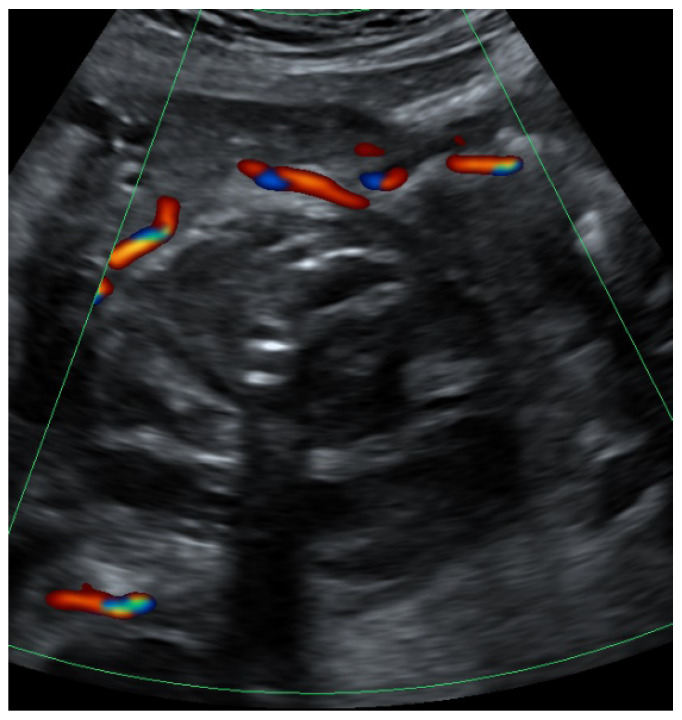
Color Doppler showing the findings 6 days after angiographic coil embolization with absence of intralesional flow, change in echogenicity and regreening of the hematoma.
